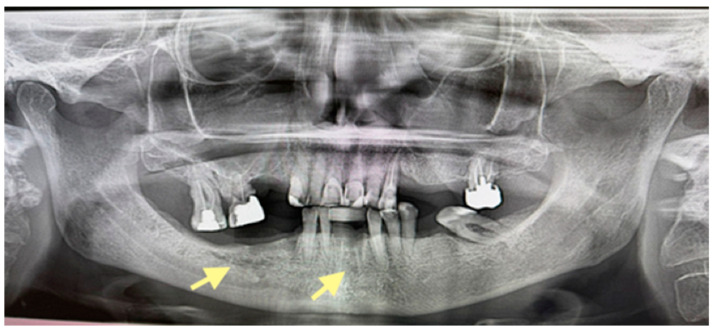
OPG (orthopantomogram) performed on 21.08.2024.
